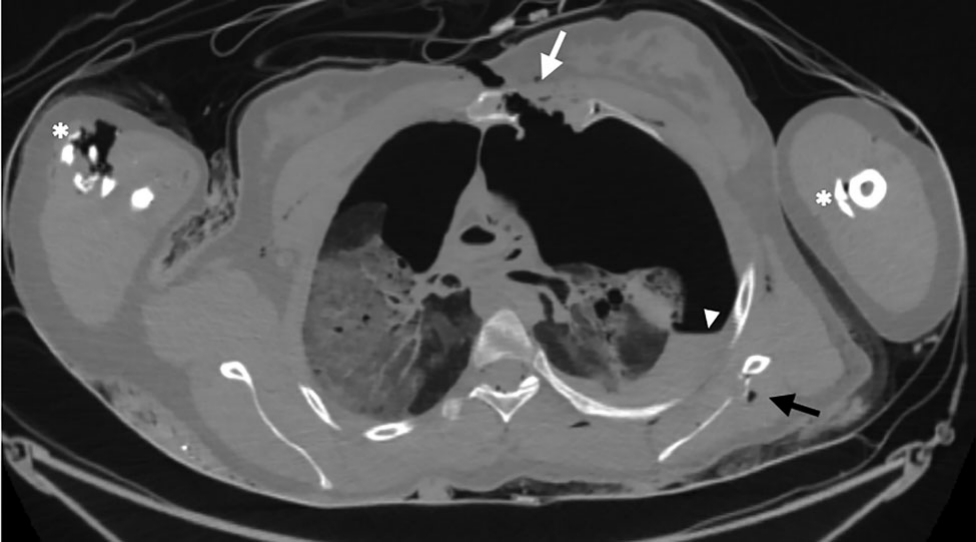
Axial postmortem computed tomography (PMCT) image at the level of the chest showing a clear intrathoracic ballistic trajectory with an anterior entry hole and diastasis of the presternal skin and subcutaneous fat. Note the perforation of the sternum anteriorly (white arrow) and the perforation of the left scapula posteriorly (black arrow) with outward-directed bone fragments indicative of the direction of the projectile. Left pneumothorax with a hyperdense liquid is visible posteriorly (arrowhead), indicative of haemorrhage in the thoracic cavity. There are also fractures of the left and right humerus (asterisks).
